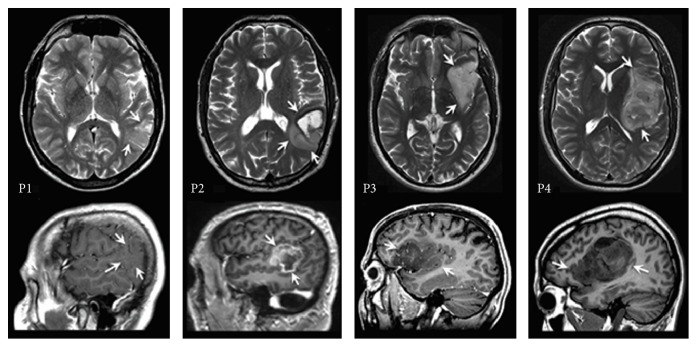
Preoperative MRI scans axial T2 weighted and sagittal T1 weighted (contrast-enhanced in P1 and in P3) sections depicting tumor localization (arrows).
